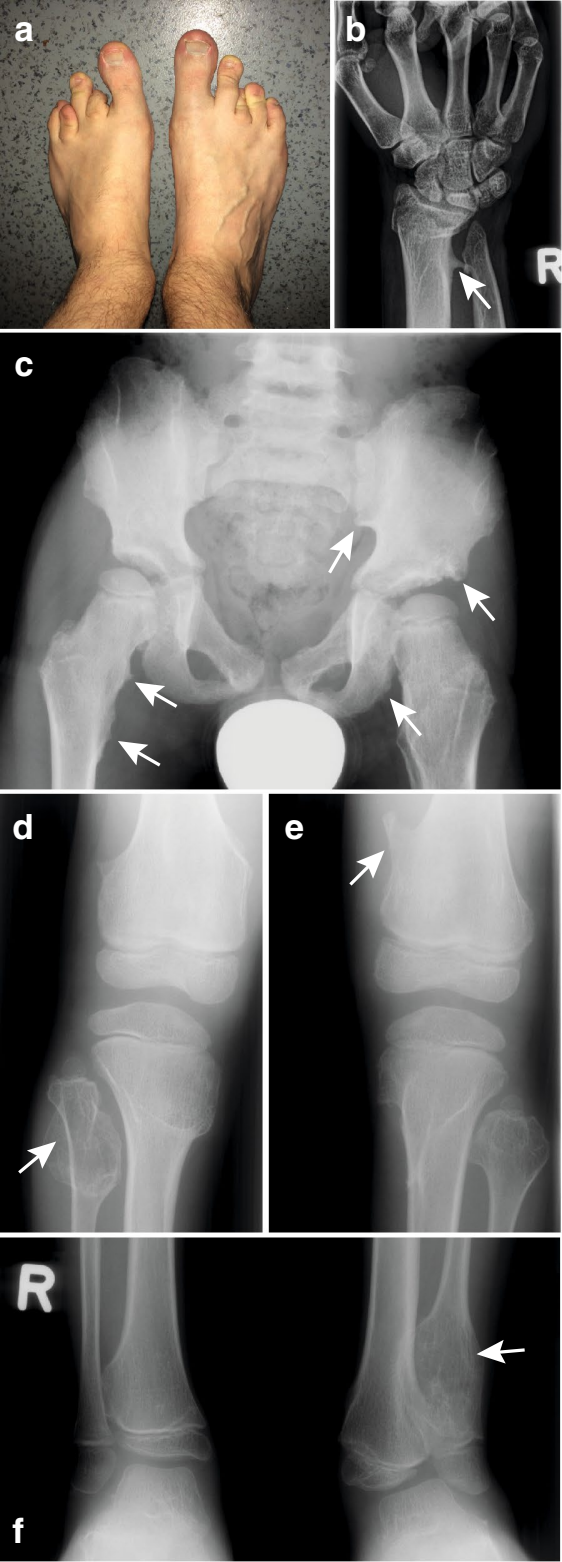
Phenotype of patient #13. A picture of the feet (a; age 34) shows unequal length of toes. An X-ray image of the right hand (b; age 19) shows an exostosis of the distal radius (*arrow*) and a shortened ulna. The X-ray of the pelvis (c; age 6) exhibits multiple exostoses on the pelvis and on the proximal femurs, which cause a locally irregular, tubercular aspect of the bone surface (*arrows*; compare the indicated regions with the contralateral side). The X-ray of the right knee (d; age 6) shows an osteochondroma on the head of the fibula (*arrow*). The X-ray of the left knee (e; age 6) demonstrates a prominent exostosis on the medial part of the femur (*arrow*). The X-ray of the lower legs (f; age 7) shows an osteochondroma at the distal end of the left fibula
